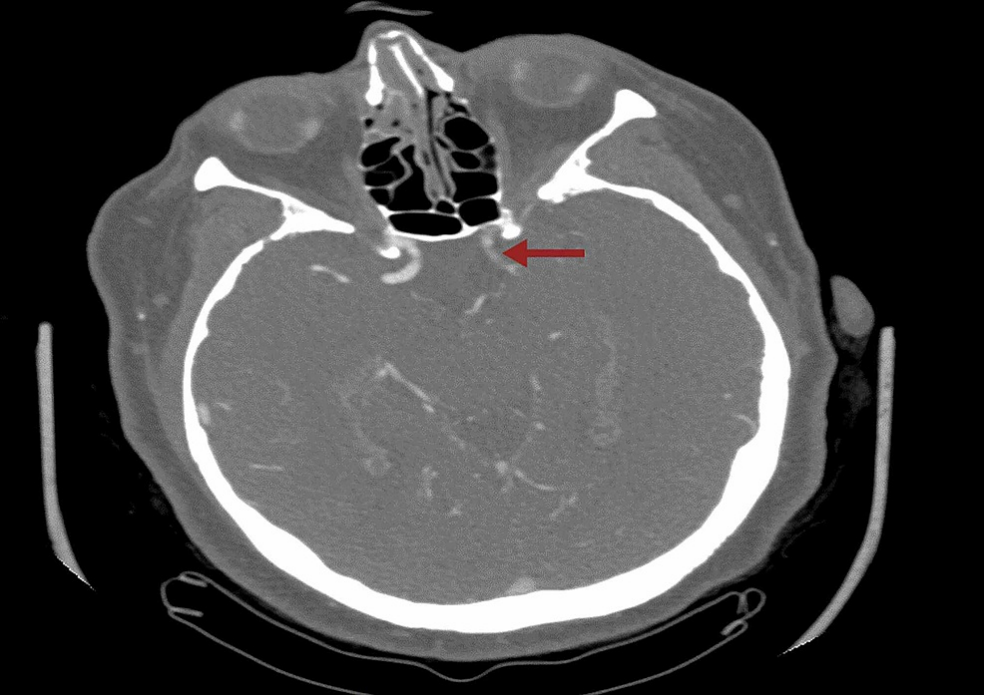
CT perfusion study showing occlusion of the supraclinoid segment of the left ICA. ICA: internal carotid artery.
